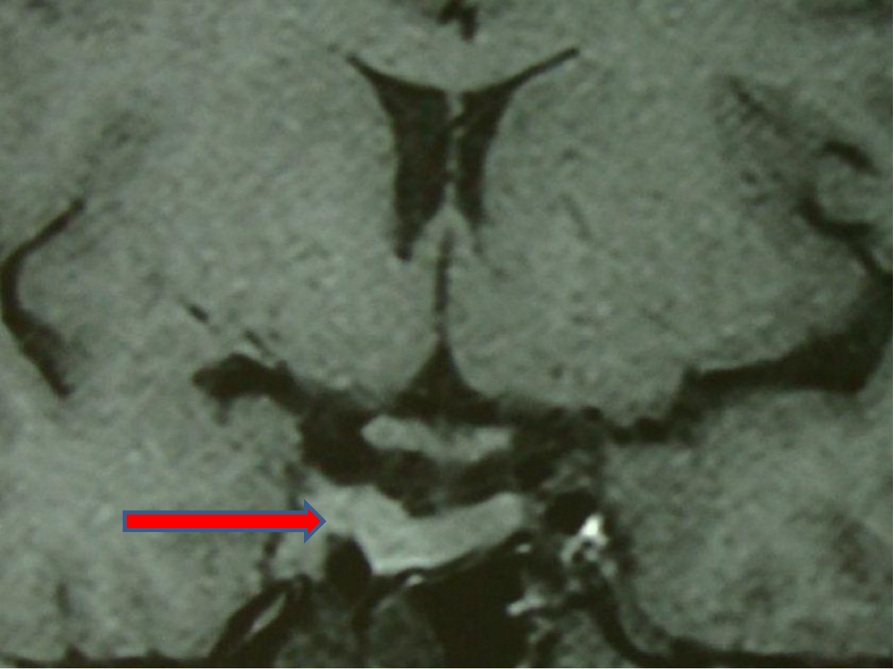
Repeat MRI (T1 weighted image) in the second trimester during pregnancy was done in order to assess the tumor size. It demonstrates considerable reduction in the tumor size, pituitary mass now being 1.8 × 1.0 cm (marked with a red arrow) as compared to the previous MRI shown in Figure 1.
